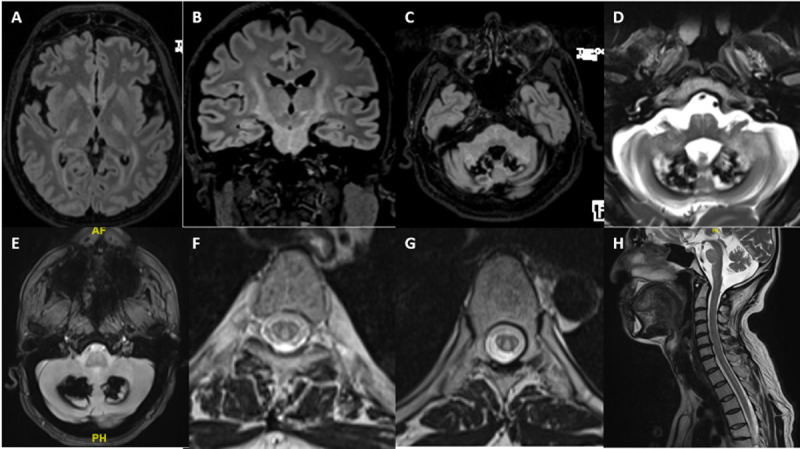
MRI of Patient-2. FLAIR axials (A, C), coronal (B) and T2 axial (D) showing symmetric hyperintensities along periventricular white matter, corticospinal tracts, middle cerebellar peduncle, dentate and peri-dentate white matter, and along inferior olives. “Hot cross bun” sign can be seen within pons (C). Susceptibility-weighted imaging (E) showing blooming in dentate nuclei. Long segment hyperintensity of dorsal and lateral columns noted in the axial (F, G) and sagittal (H) T2 sequences of MRI spine.
